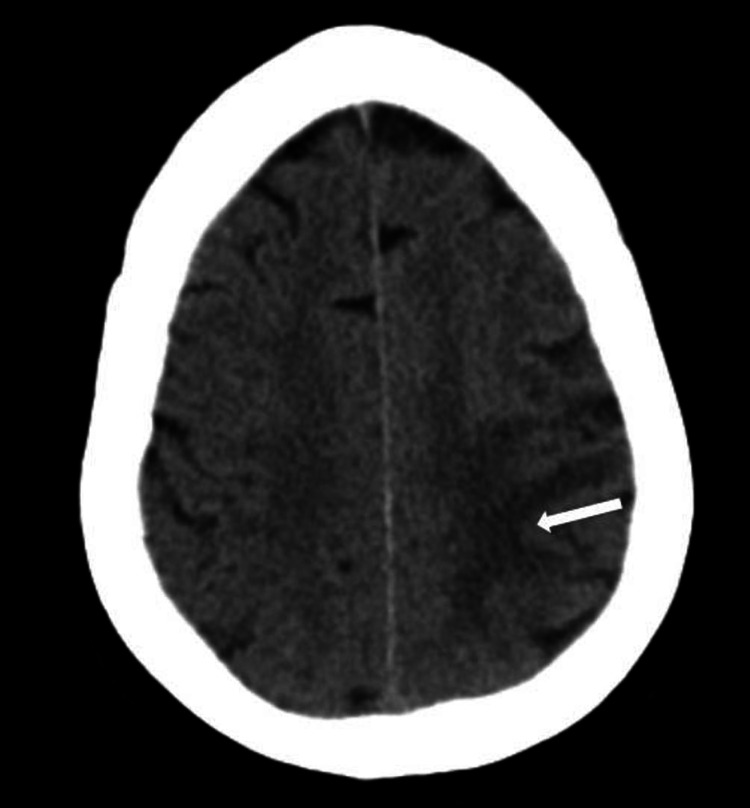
CT Head without contrast, demonstrating subacute infarct and cerebral edema (arrow) CT: computed tomography
